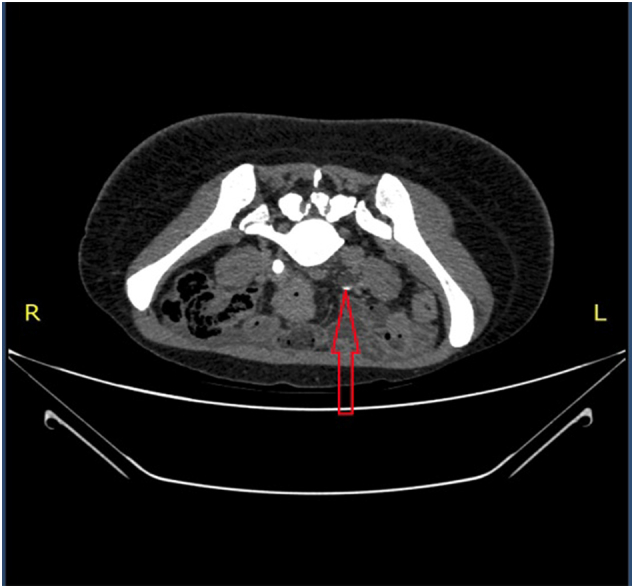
CT scan of the abdomen with IV contrast in prone position – bilaterally enlarged ovary with masses within and distal left ureteric narrowing.
